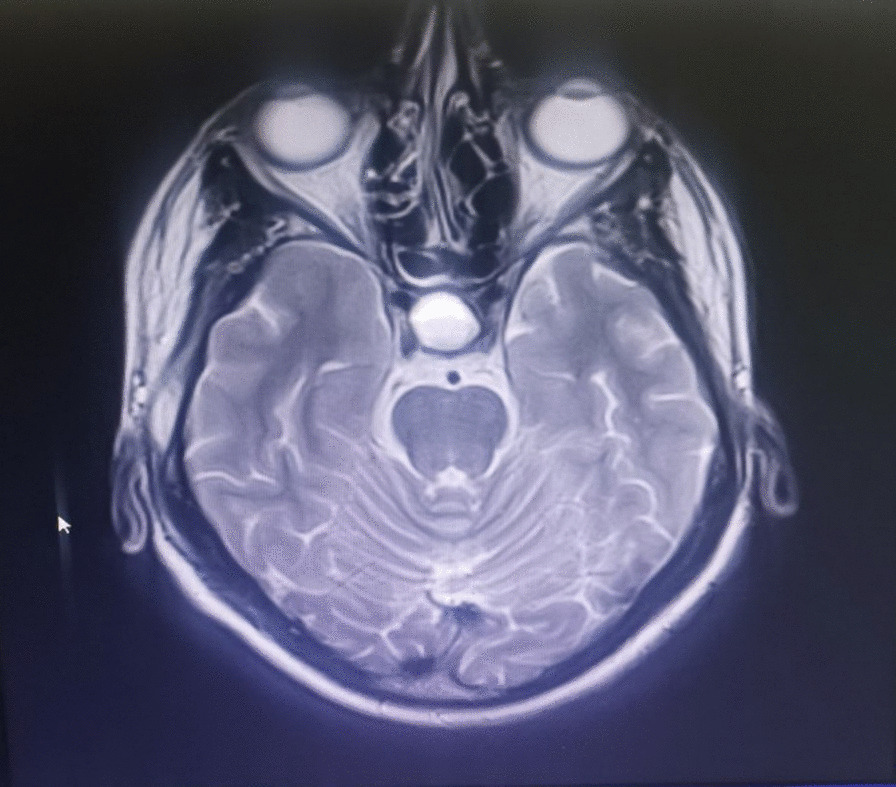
MRI of the brain/Coronal section
